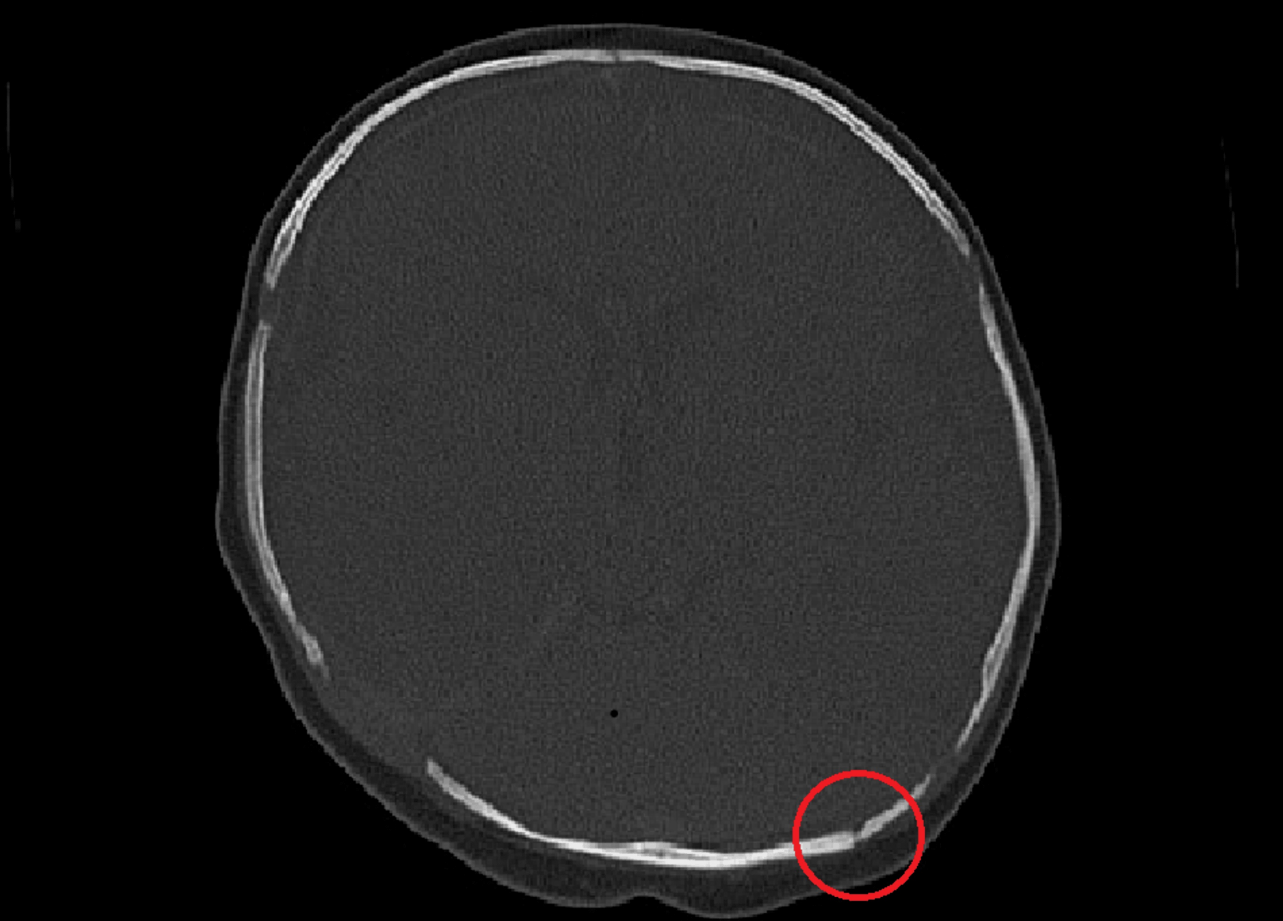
Non-contrast head CT, axial section, bone window. Squamous part of the occipital bone: fracture line on the left side (red circle); asymmetry of the lambdoid suture.
